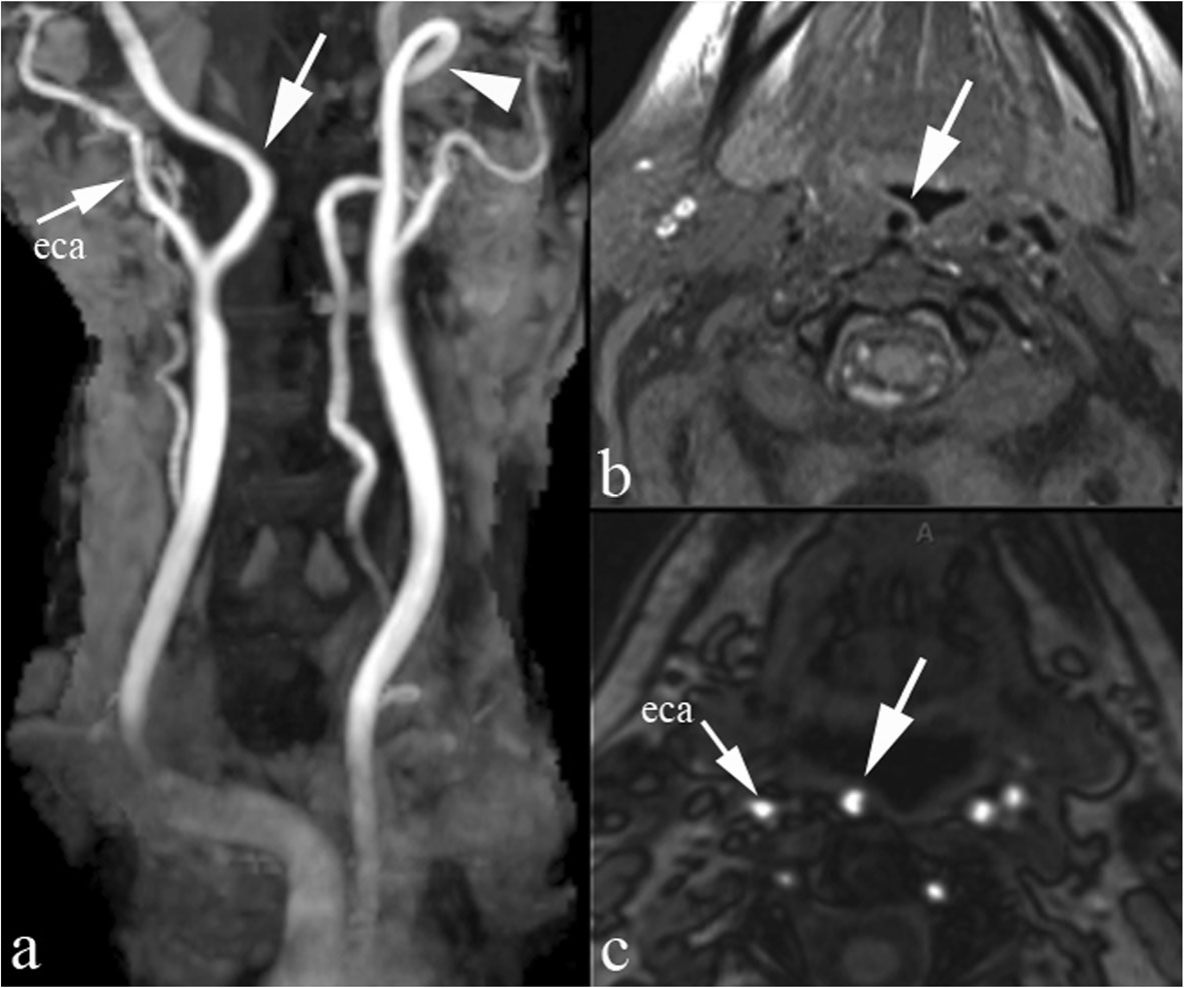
Retropharyngeal carotid artery*.* MRI 3D TOF, thick maximum intensity projection (MIP) reconstruction on coronal plane (a) and native axial partition (c); fat suppressed axial SE T1 with contrast administration (b). The right ICA (large arrows) bends medially reaching the midline behind the posterior oropharyngeal wall. Coiling of the left ICA (arrowhead). eca external carotid artery
